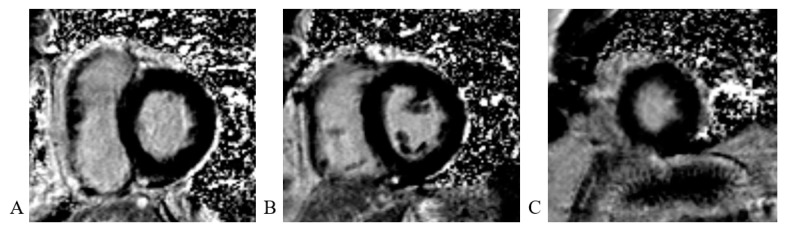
Cardiac magnetic resonance imaging: (A) LGE PSIR sequence, short axis, basal view; without visible zones of LGE phenomenon; (B) LGE PSIR sequence, short axis, mid chamber view; without visible zones of LGE phenomenon; (C) LGE PSIR sequence, short axis, apical view; without visible zones of LGE phenomenon.
